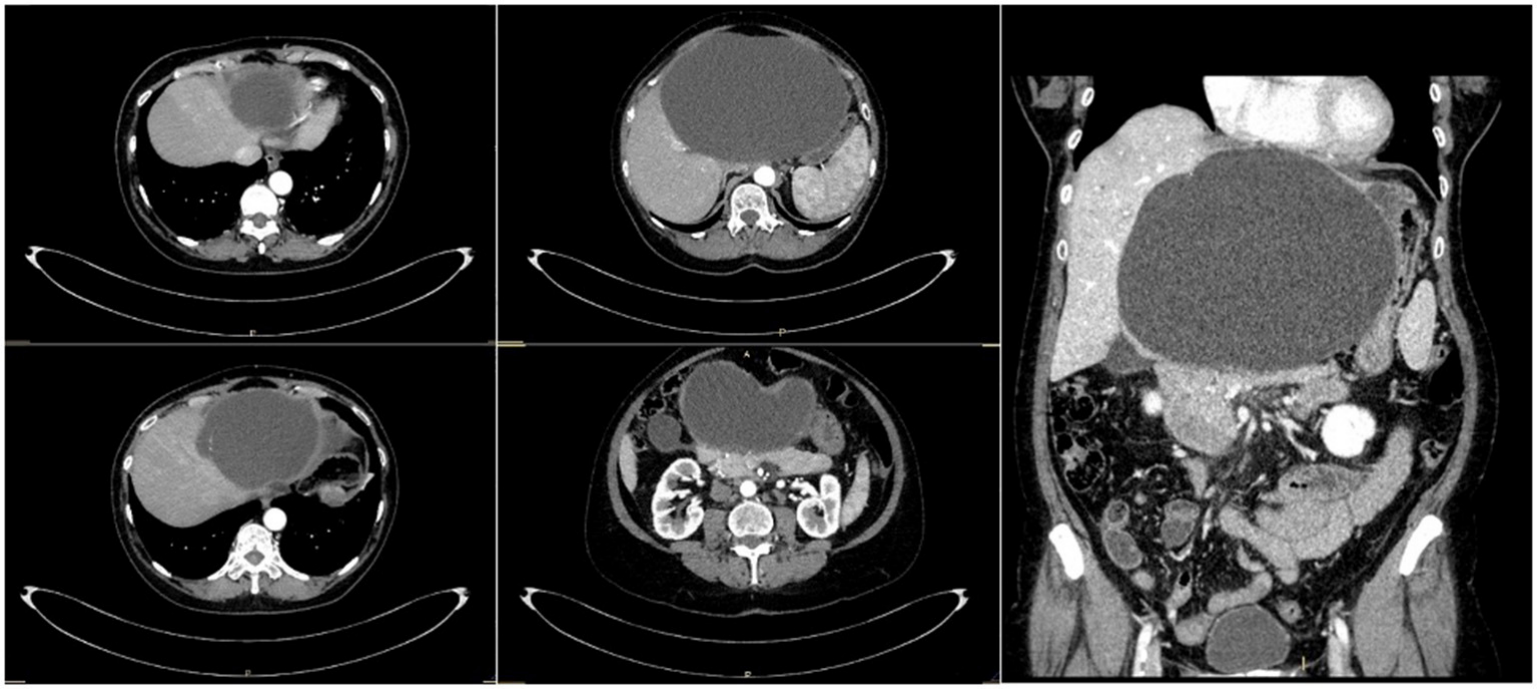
CT scan of Patient 1 showing the liver cyst that occupies all the left hemi-liver.
